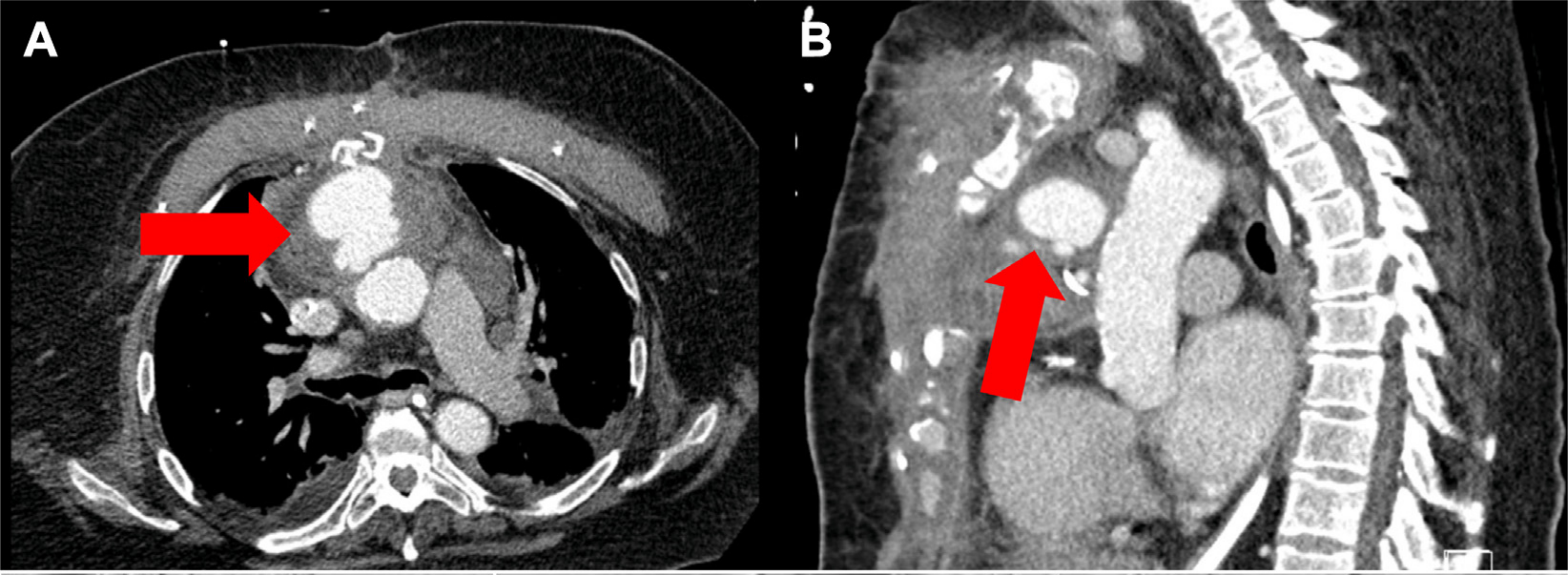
Preoperative Contrast Enhanced Computed Tomography Angiography Preoperative contrast-enhanced computed tomography angiography shows the ascending aorta zone 0 pseudoaneurysm (5 cm × 5 cm × 5 cm) arising in the saphenous vein graft anastomosis in both axial (A) and sagittal (B) views.
